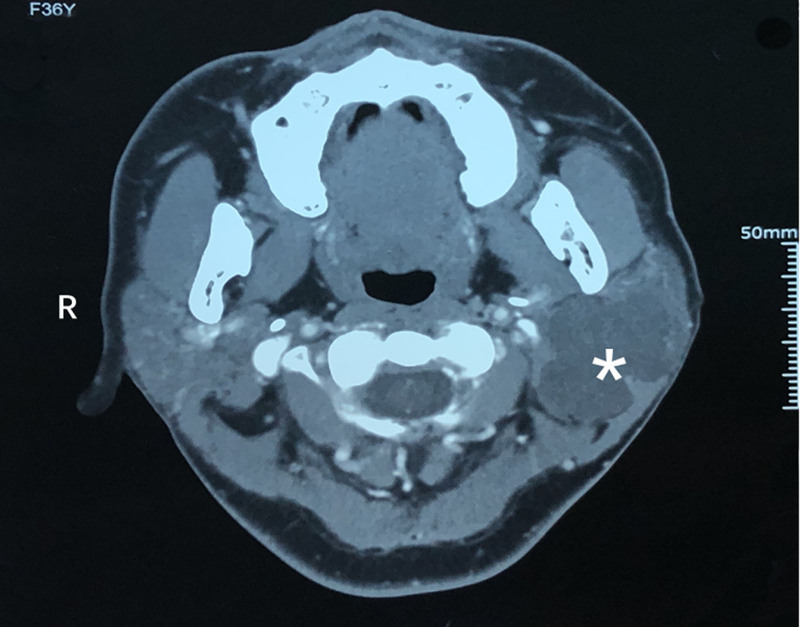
Enhanced CT revealed a nodular lump (*) in the parotid gland on the left side. CT = computed tomography.
